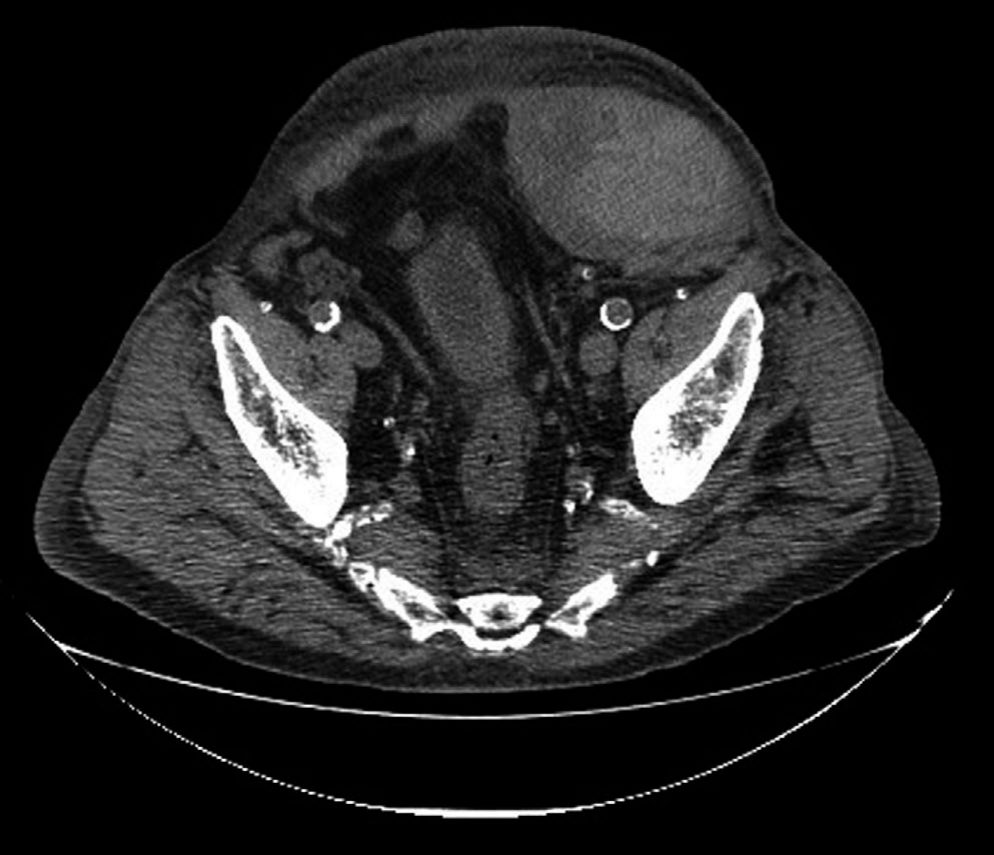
Case 3: CT scan of the abdomen and pelvis revealing a left rectus sheath hematoma. Heterogeneous thickening of the left large rectus abdominis muscle, with dense areas, revealing a hematoma with still relatively recent hemorrhage
